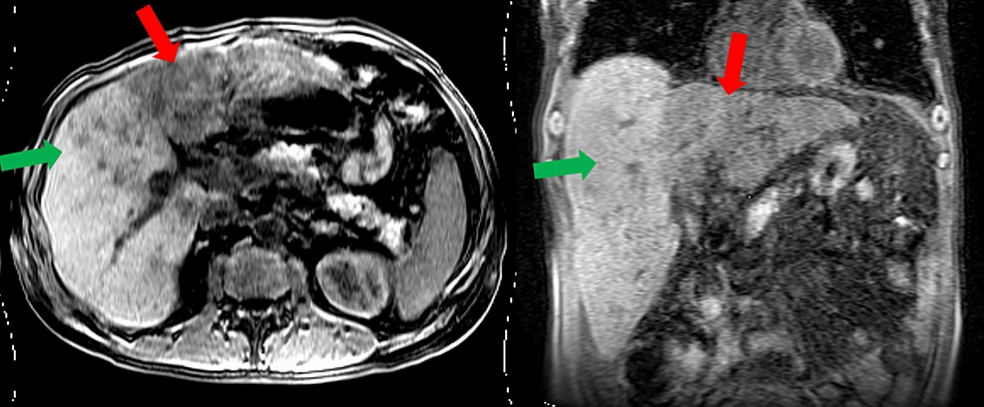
Magnetic resonance imaging of the liver in axial (left) and coronal (right) sections showed liver cirrhosis with widespread signal abnormality throughout the liver. Infiltrative hepatocellular carcinoma of the left and caudate lobes (red arrow) with intrahepatic metastases to the right lobe (green arrow) not excluded per radiology report.
